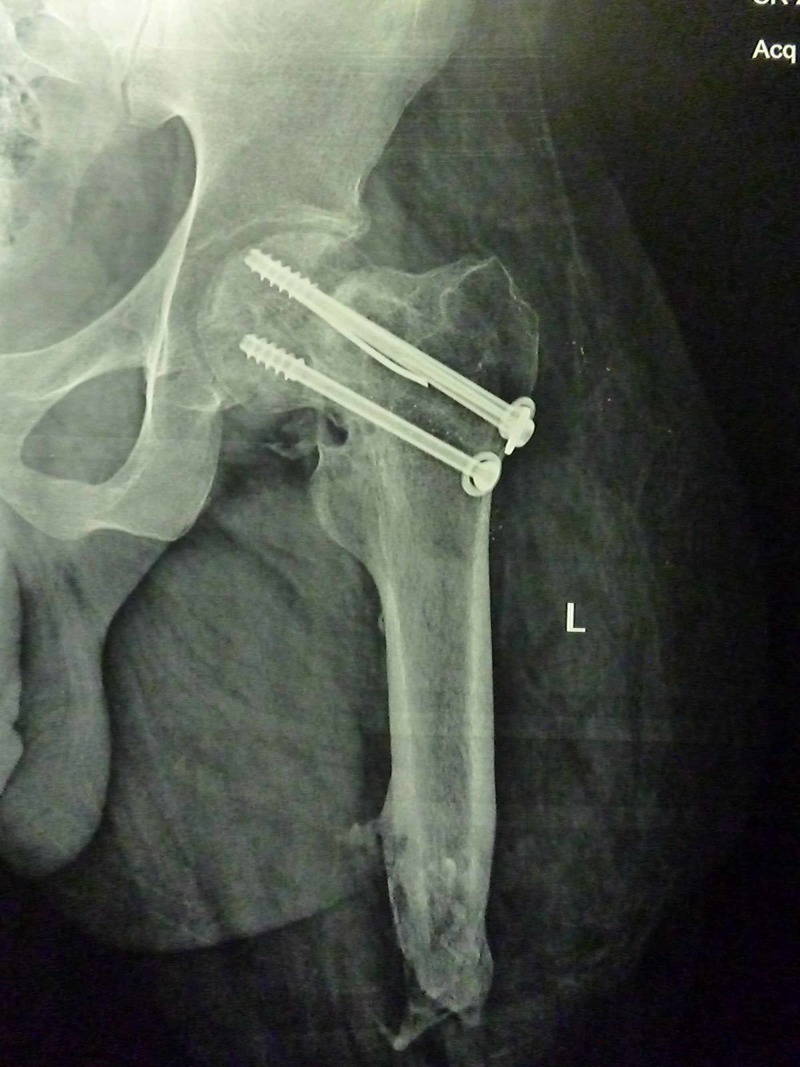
One year follow up antero-posterior radiograph of the patient A showing healed fracture and incorporated fibula. Also note the broken guide-wire which has not migrated.
